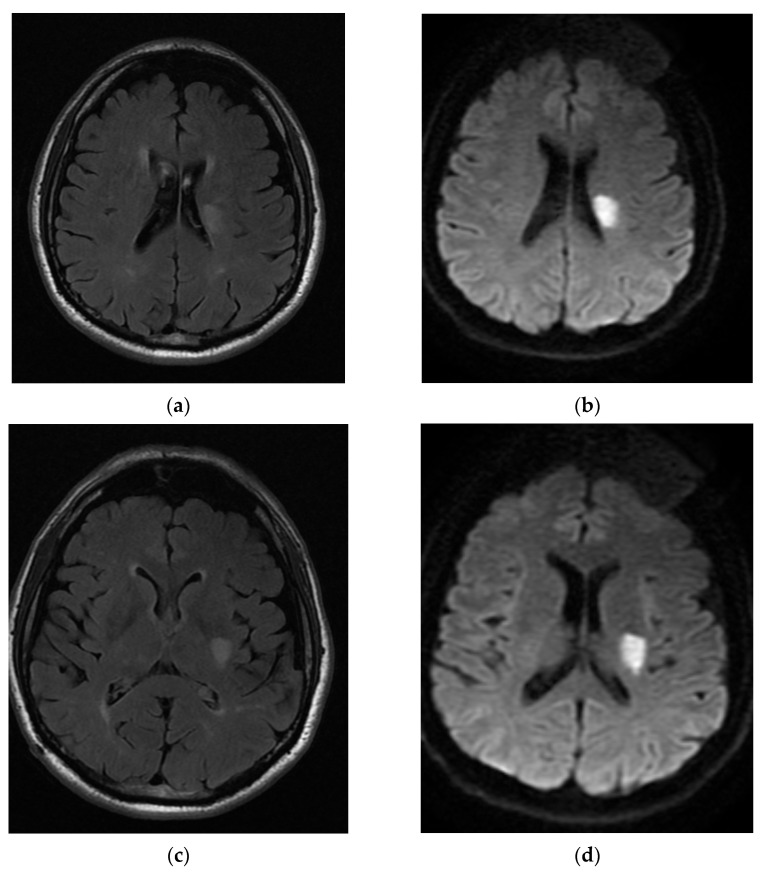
The patient’s brain MRI: axial flair (a,c) and DWI (b,d), with an acute ischemic lesion involving the left anterior choroidal artery. Also shown is ischemic microangiopathy.
